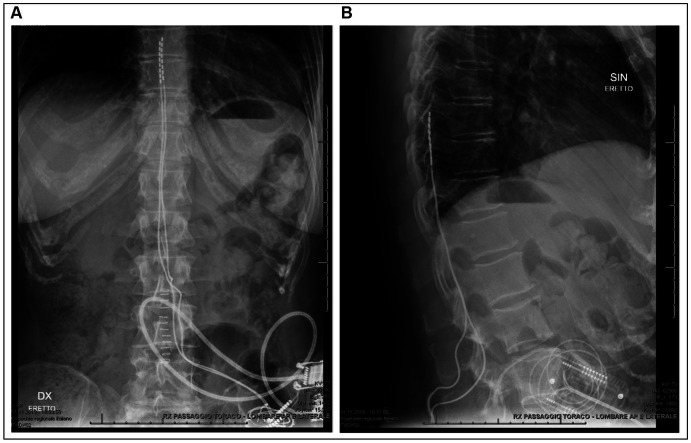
Radiography of patient 1 after definitive implantation confirming the midline position and spinal level of the lead electrodes. (A) Anteroposterior chest and abdominal radiography. (B) Lateral radiography of the thoracic and lumbar spinal column.
